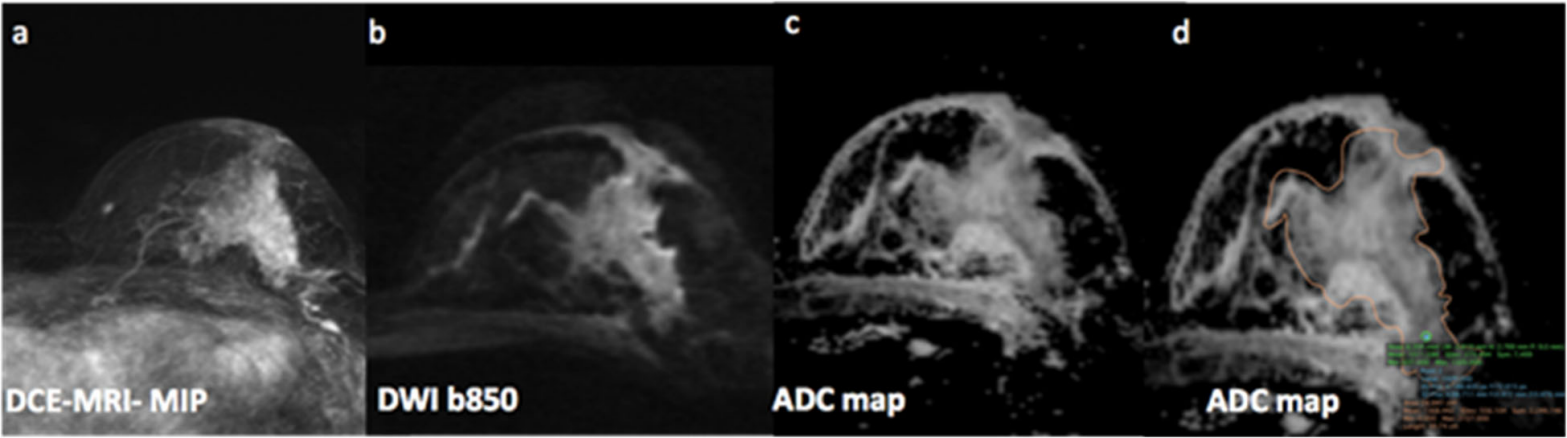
Invasive lobular cancer in a 49-year-old, with biopsy-proven invasive lobular cancer, grade 2. a Dynamic contrast-enhancement MRI maximum intensity projection of the left breast shows in the upper-outer quadrant, a 63-mm segmental area of non-mass enhancement, with heterogeneous internal enhancement pattern. b Diffusion-weighted sequence at b 850 and c apparent diffusion coefficient (ADC) map. d Among the three segmentation tools, the darkest part of the tumor (Dptu) 2D region of interest (ROI) proved to yield the highest diagnostic accuracy, showing the lowest ADC values (Dptu ADC mean, 1.021 × 10^−3^ mm^2^/s; whole tumor ROI, 1.568 × 10^−3^ mm^2^/s; point tumor delineation ROI, 1.029 × 10^−3^ mm^2^/s)
